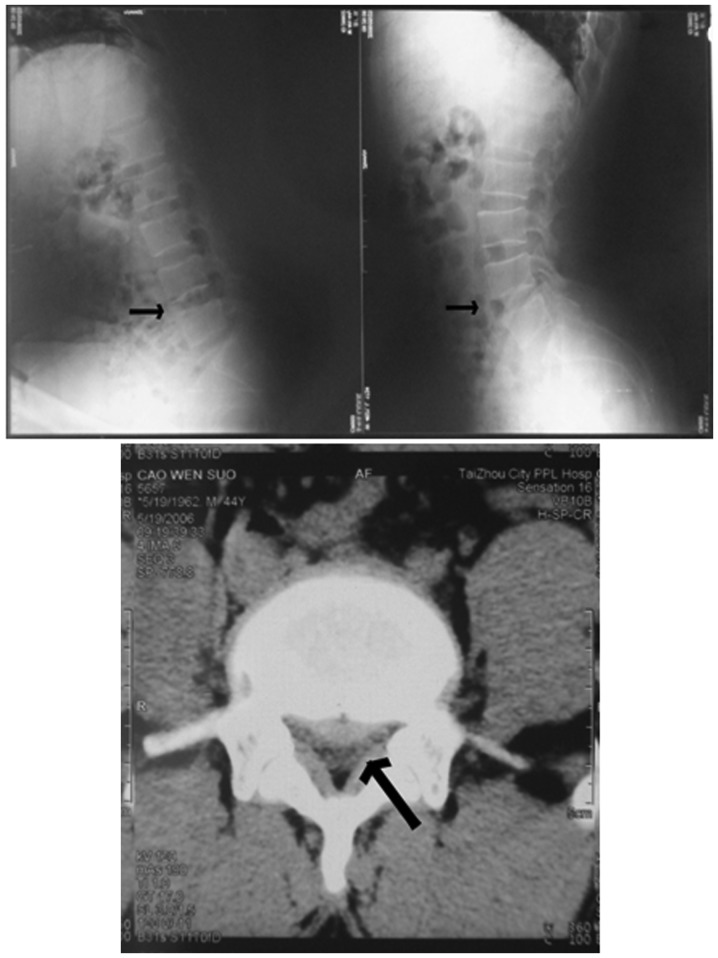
Male, 53-year-old patient with chronic lumbosacral pain for >5 years. Preoperative lumbar dynamic topography (black arrow) suggested vertebral instability between waist 4 and waist 5. Lumbar computed tomography (CT; black arrow) suggested a prolapsed intervertebral disc between waist 4 and waist 5, which clearly compressed the dural sac.
